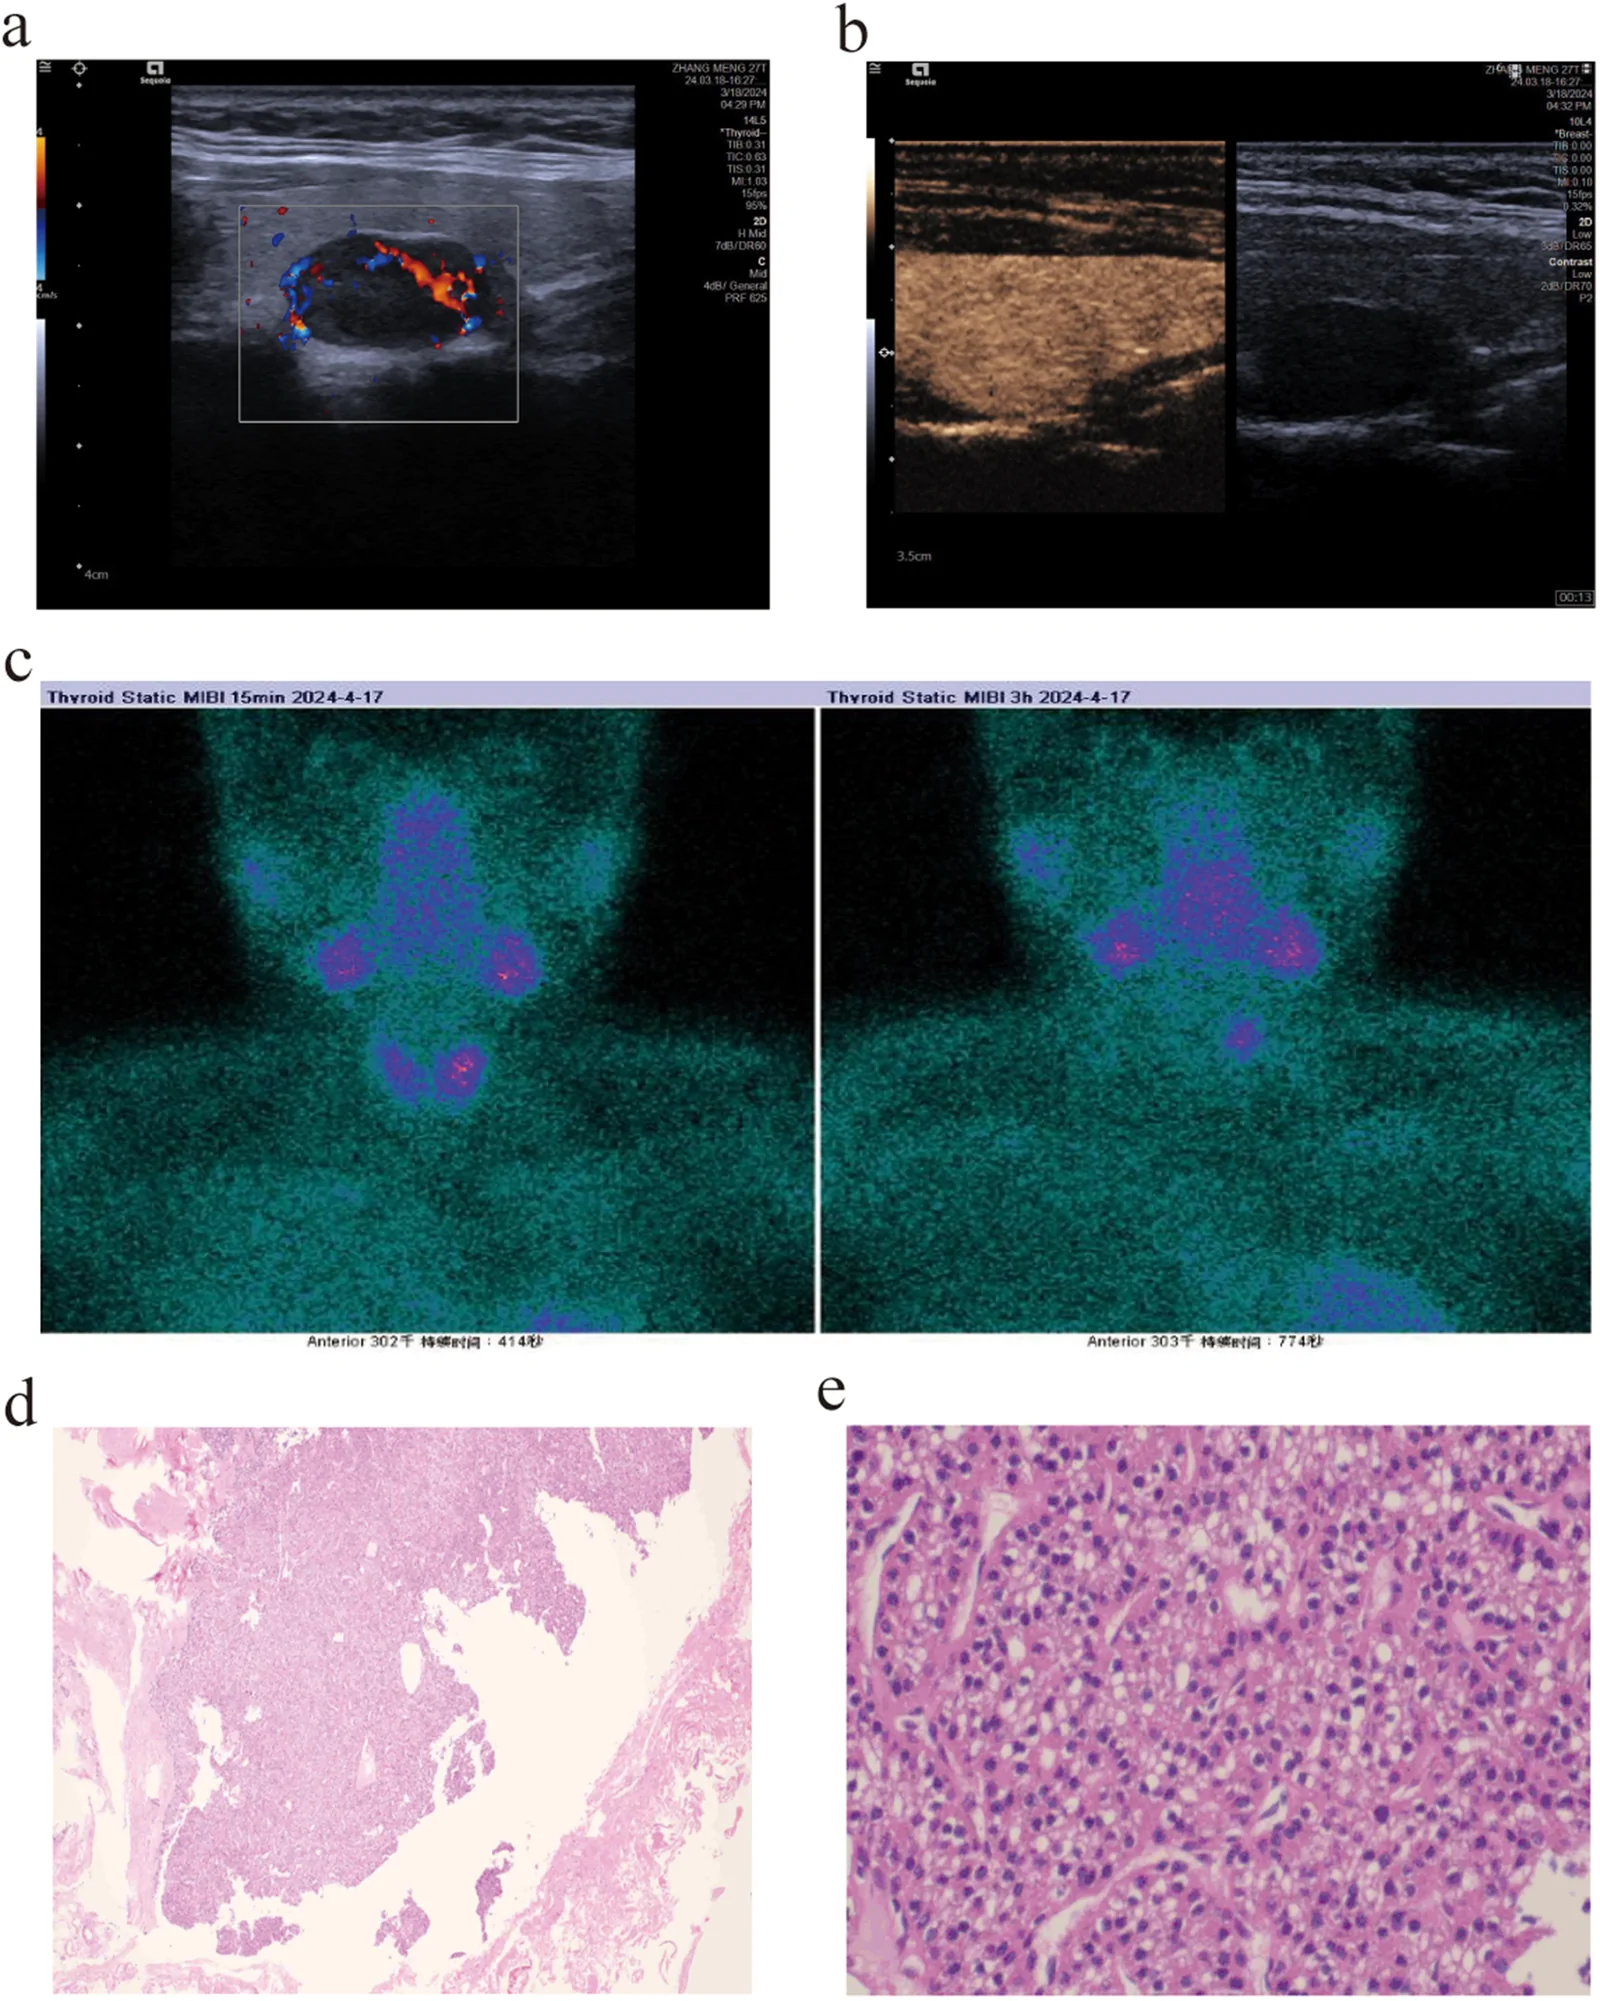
(a,b) Thyroid ultrasound. (c) ECT - ectopic parathyroid phenomenon. (d,e) Pathology.
